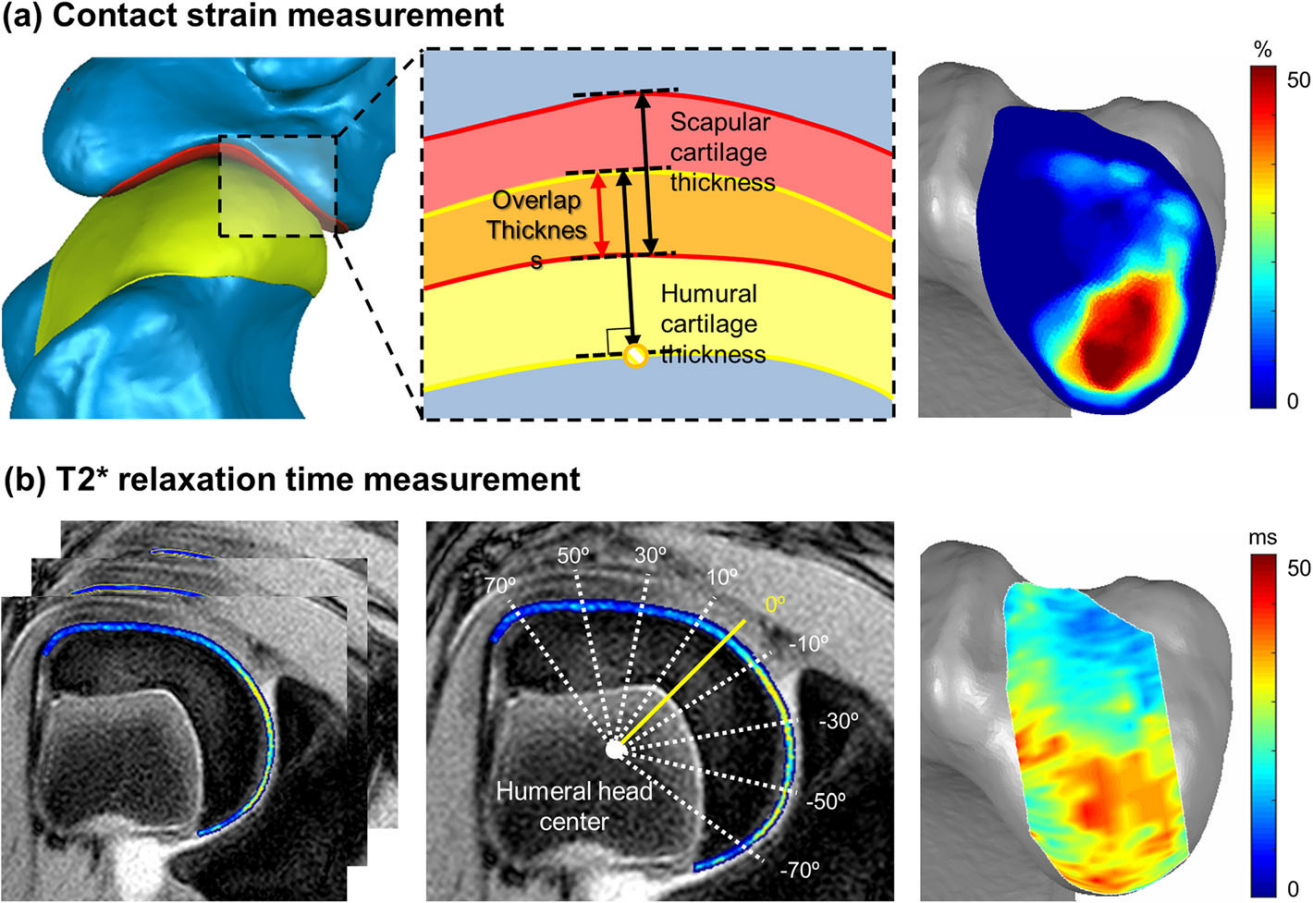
3D maps of articular cartilage contact strain and T2* relaxation time. a Articular cartilage contact strain was defined as the ratio between the undeformed articular cartilage thickness and the thickness of the overlapping areas between the scapular and humeral articular cartilage. b The T2* relaxation time of each pixel in the MR images was calculated and each articular cartilage surface was divided into multiple anterior-to-posterior 20° regions
